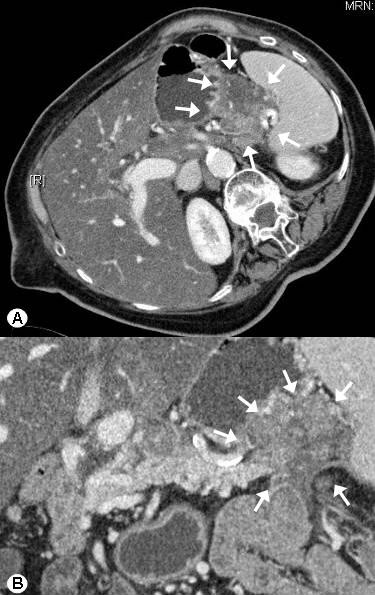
Computed tomography scan of the abdomen revealed a large lesion of 5 to 6 cm in relation to the pancreatic body and tail (venous phase A, B, arrows).
